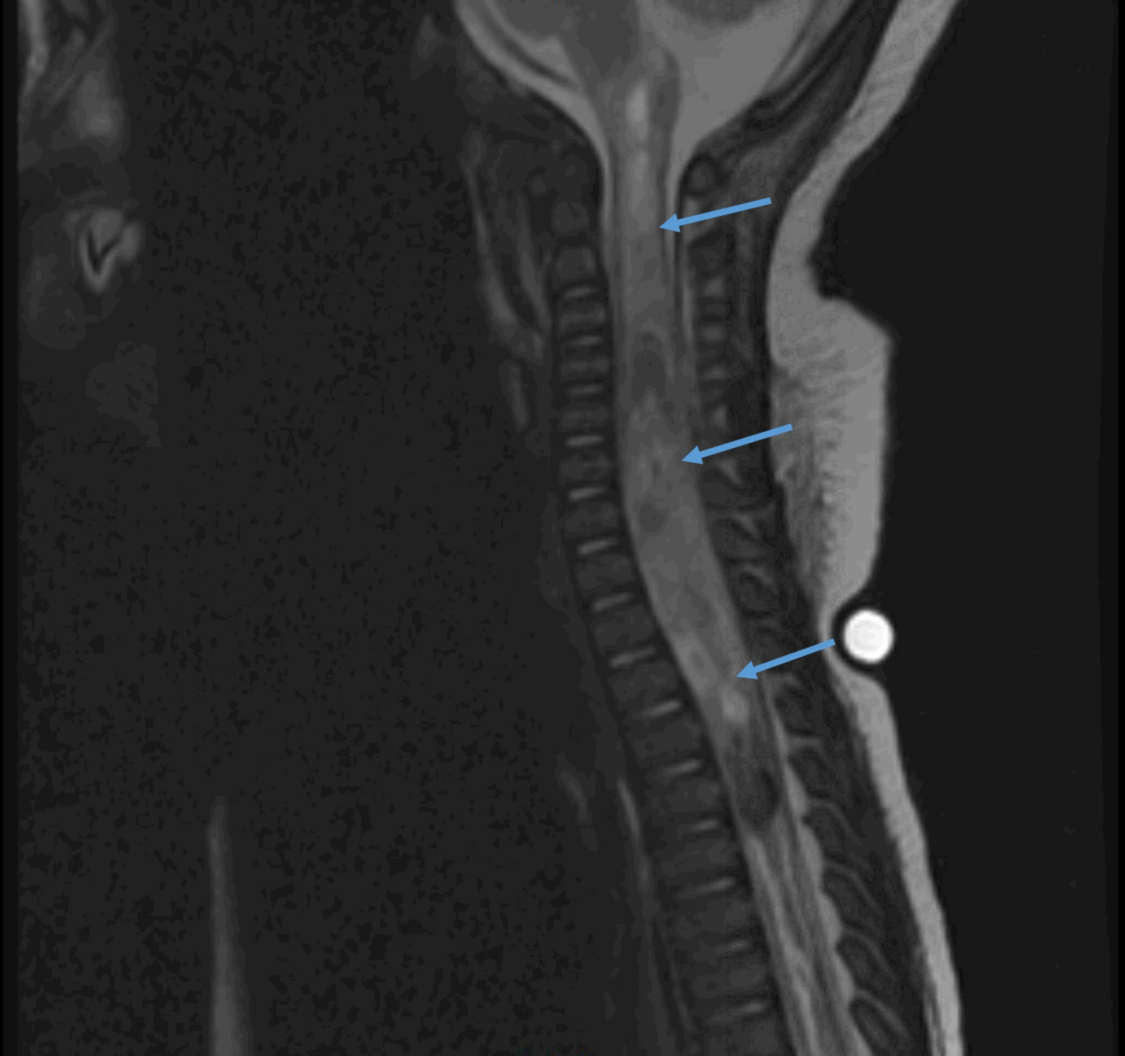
MRI Spine. MRI of cervical, thoracic, and lumbar spine demonstrating a near holocord hemorrhagic, intramedullary mass consistent with a congenital immature teratoma. MRI: Magnetic resonance imaging
